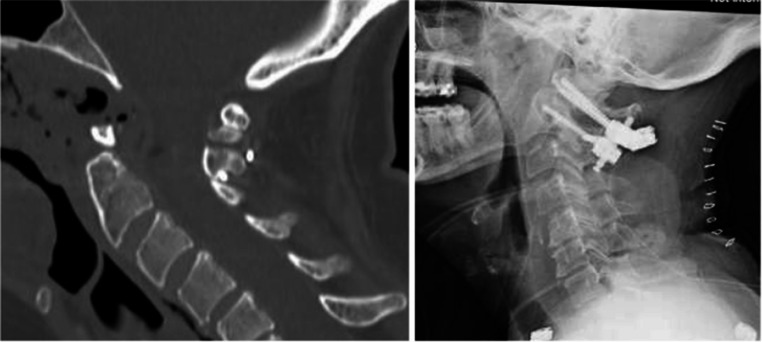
CT imaging anf plain film at three weeks post-op demonstrating staisfactory decompression and placement of hardware
